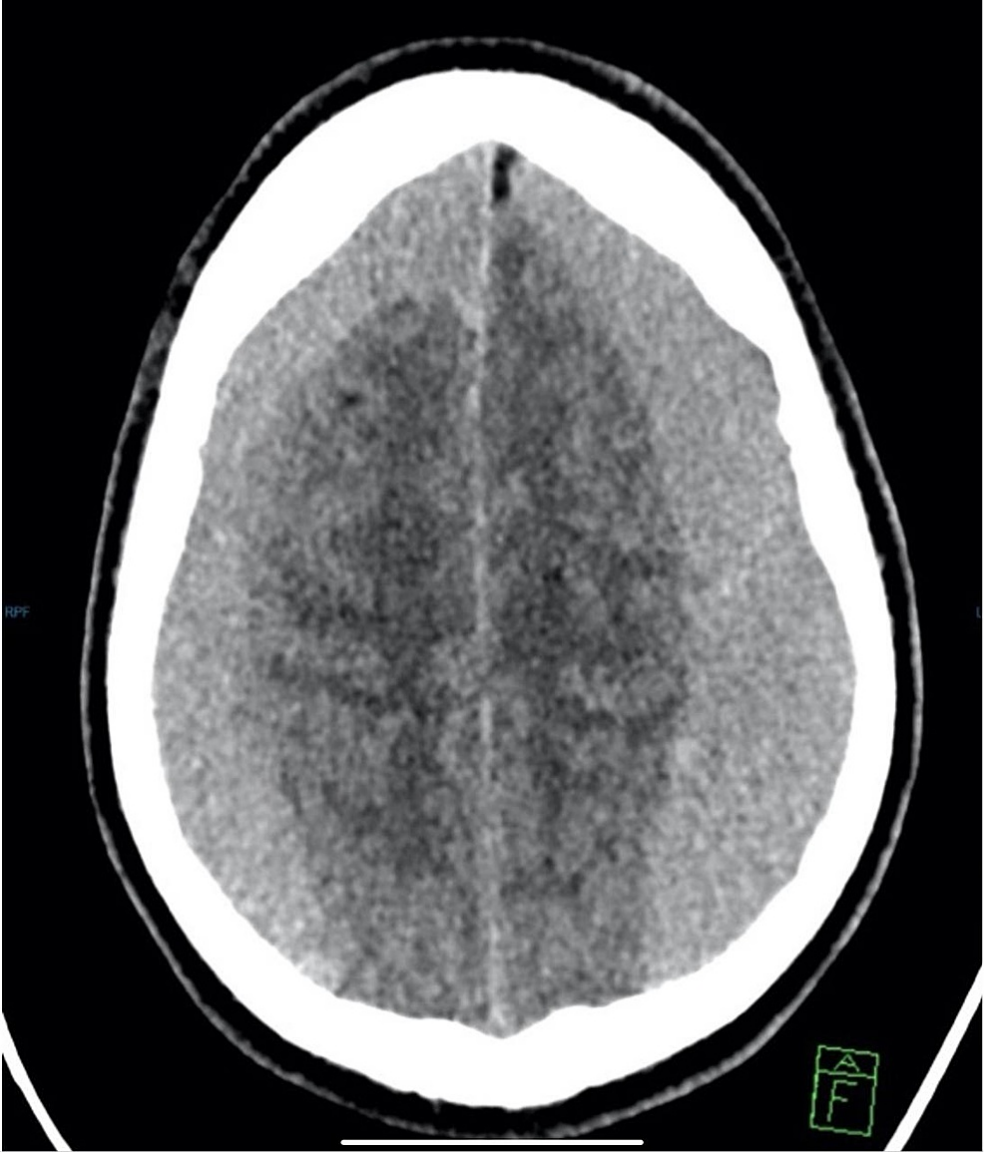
CT brain axial view: significant bilateral subacute subdural hematoma with significant mass effect on the brain cortex with effacement of the sulci and brain edema.
